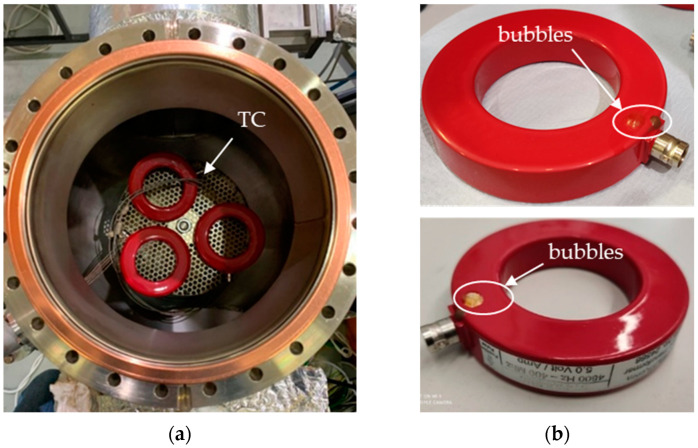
Magnelab CT: (a) in a vacuum chamber; (b) sample n° 3 (M3) after bake-out at 90 °C.
